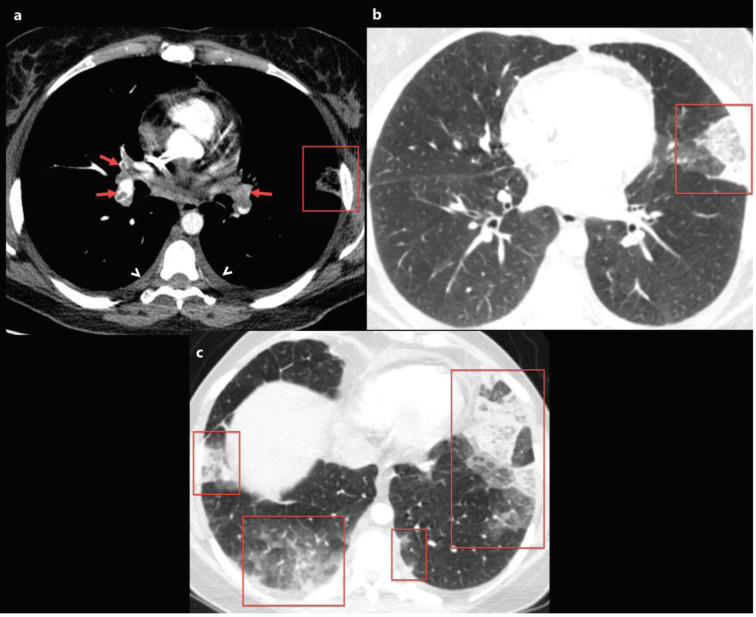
A 40-year-old female patient was presented with complaints of fever that had been ongoing for 5 days, and newly emerged severe dyspnoea. The patient’s D-dimer level was found to be very high and contrast-enhanced pulmonary CT angiography was obtained. a) Axial CT image at the level of the origin of the middle lobe bronchus with mediastinum window settings shows bilateral diffuse pulmonary embolism (red arrows), bilateral mild pleural effusion, and subpleural triangular-shaped opacity with a reverse halo sign in the left lung compatible with pulmonary infarction (red frame). b, c) Axial CT images of the same patient with lung window settings show multiple subpleural consolidation and ground-glass opacity areas (red frames). The nasopharyngeal swab test of the patient was positive for 2019-nCoV.
